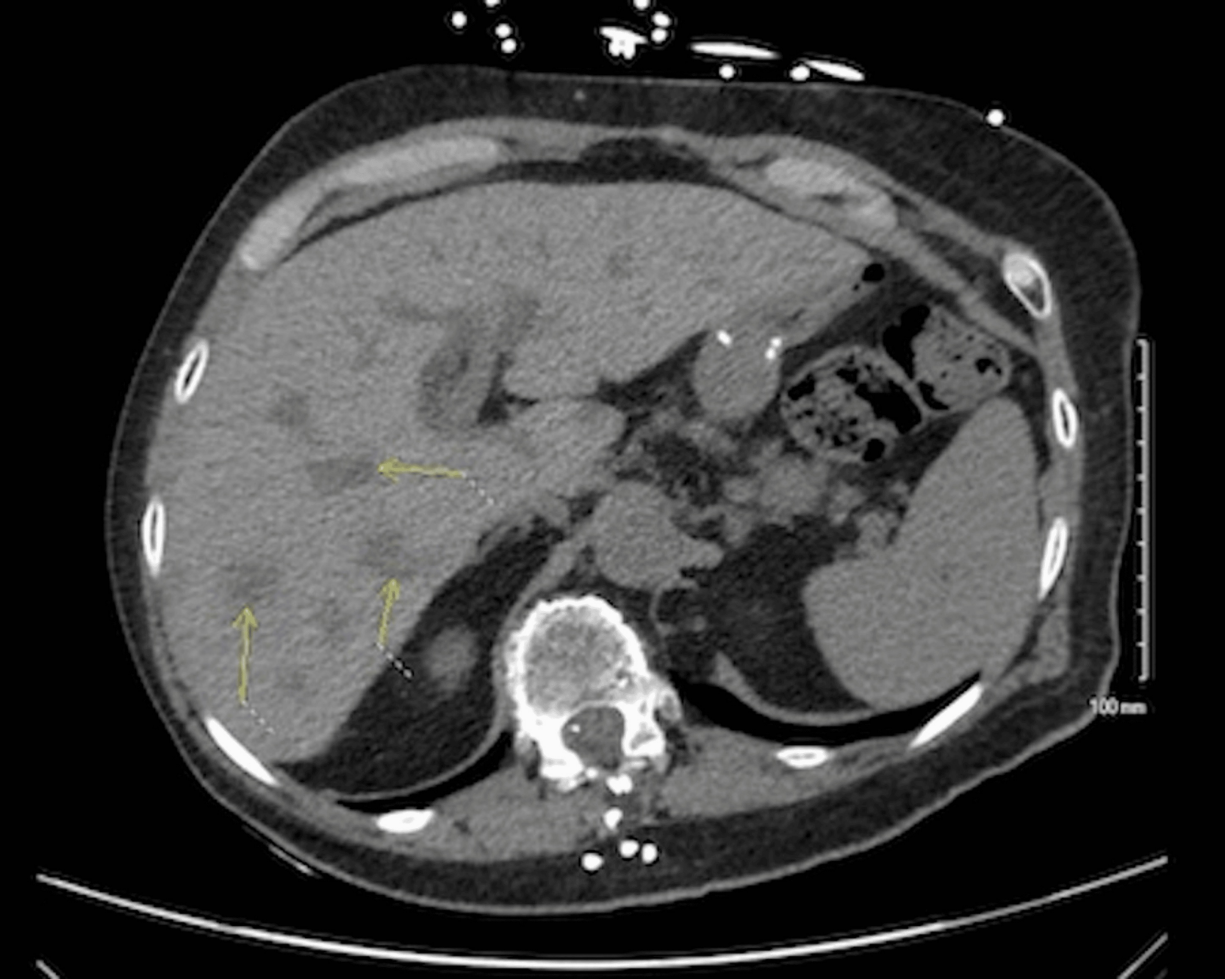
CT scan of the liver, showing multiple liver masses.
